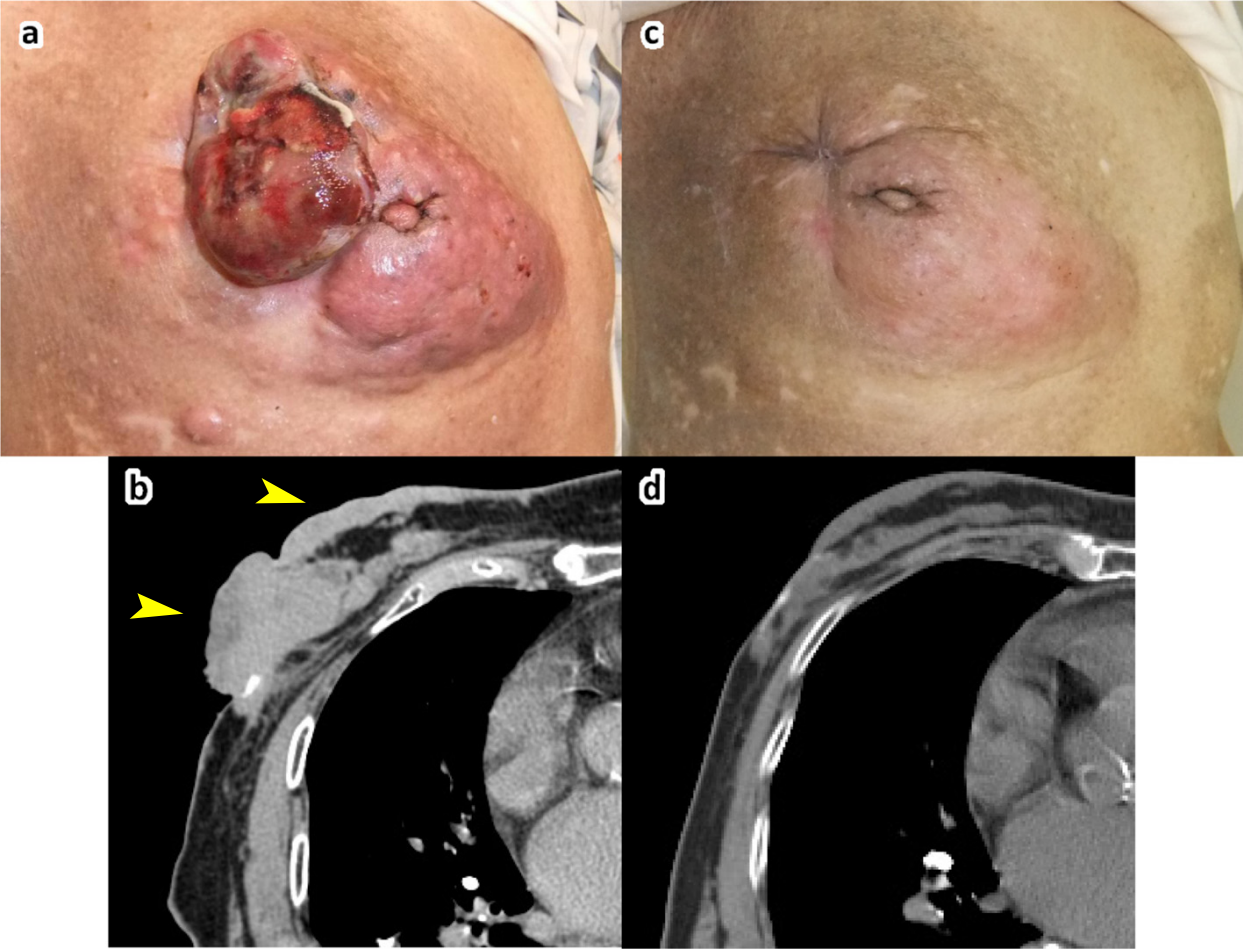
Case 1.a: Macroscopic image of the right breast at the initial visit. b: Computed tomography (CT) findings at the initial visit. CT revealed a mass with overlying and surrounding skin thickening (arrowheads). c: Macroscopic image of the right breast before surgical treatment. d: CT findings before surgical treatment.
